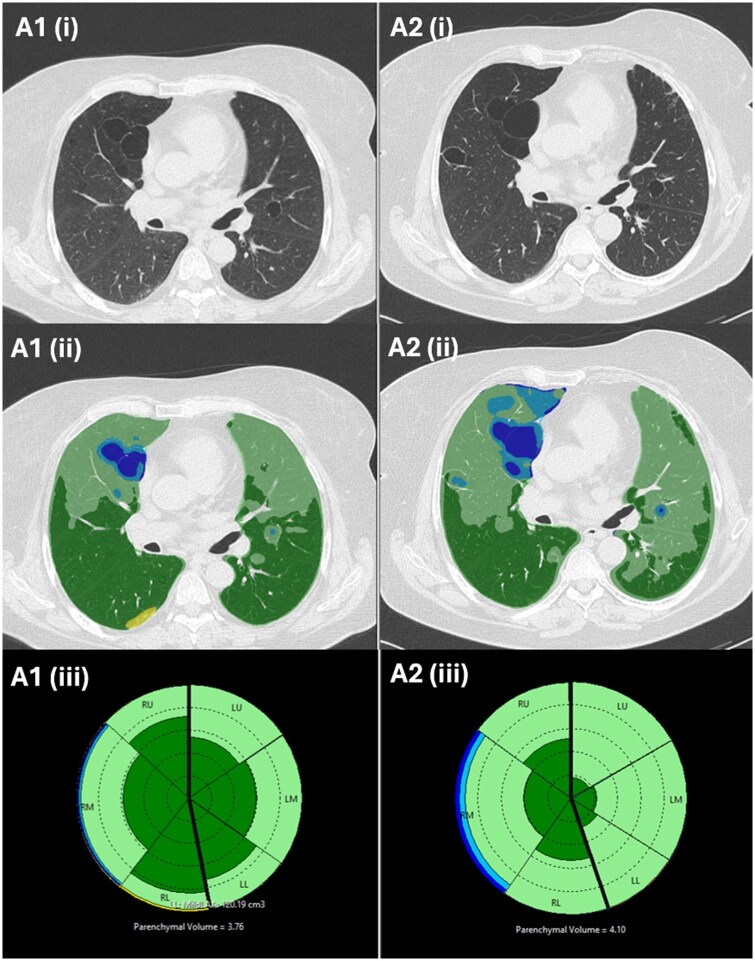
Example of CALIPER analysis of a patient with SjD-ILD. Axial HRCT slices (i), axial CALIPER-derived colour image overlays (ii) and Glyph of the lung parenchymal patterns (iii) as various colors [dark green = normal lung, light green = mild low attenuation areas (LAA), light blue: moderate LAA, blue = severe LAA] are shown. Interestingly, severe LAA accurately identify cystic areas providing an exact and reproducible measure of their extent compared with total lung parenchyma. A1 shows CALIPER evaluation at baseline with a severe LAA of 0.32%. A2 shows CALIPER evaluation at 30 months-follow-up with a severe LAA of 1.04%. The delta in the ‘severe LAA’ value, representing cystic disease progression in our case, was 0.72%
